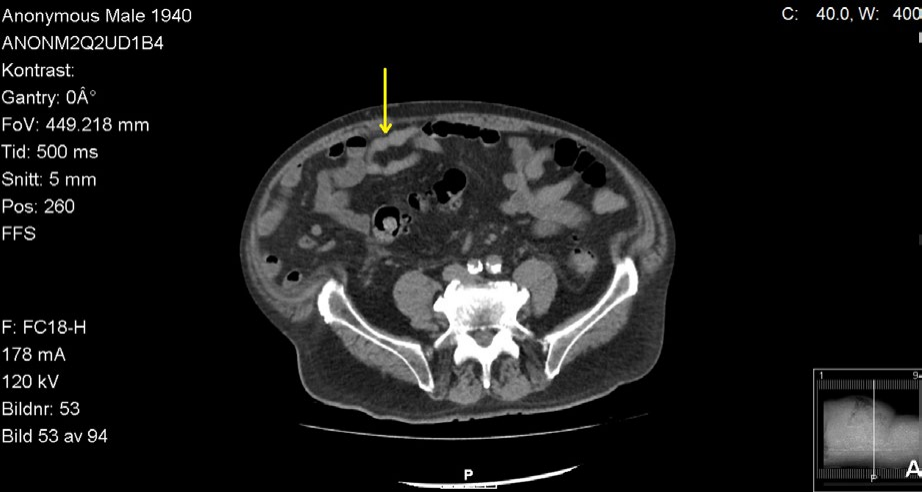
CT without contrast (axial) shows protruding paraumbilical veins
